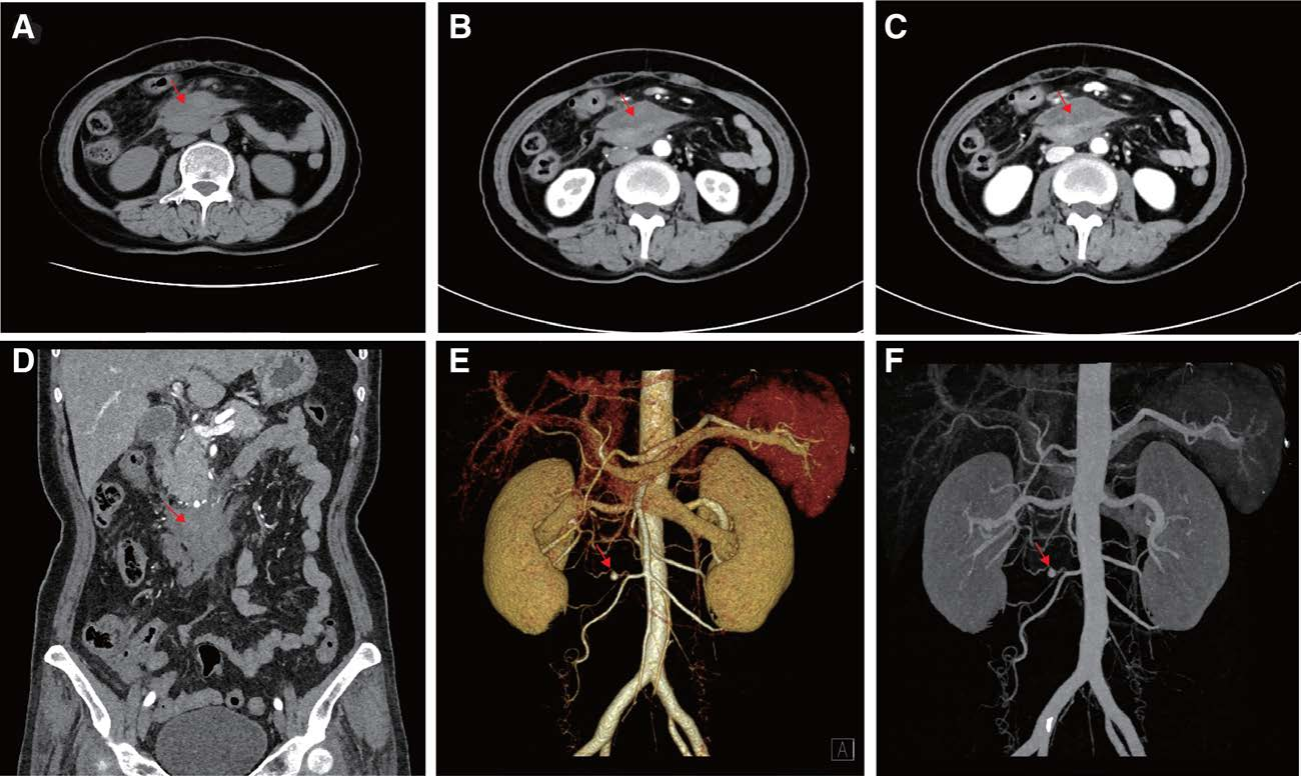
(A) nonenhanced CT shows a mass in front of the duodenum, under the head of the pancreas. The attenuation is heterogeneous, and the CT value of the patchy high-density area within the mass is 49 Hu, indicating hemorrhage. On the arterial (B) and venous (C) contrast-enhanced CT images, the mass shows heterogeneous density with no noticeable enhancement, suggesting the diagnosis of a hematoma. (D) The coronal multiplanar reformation image shows a small aneurysm above the hematoma with a diameter of 6 millimeters. The volume rendering (E) and maximum intensity projection (F) CT images show an aneurysm in the arch of the pancreaticoduodenal artery.
